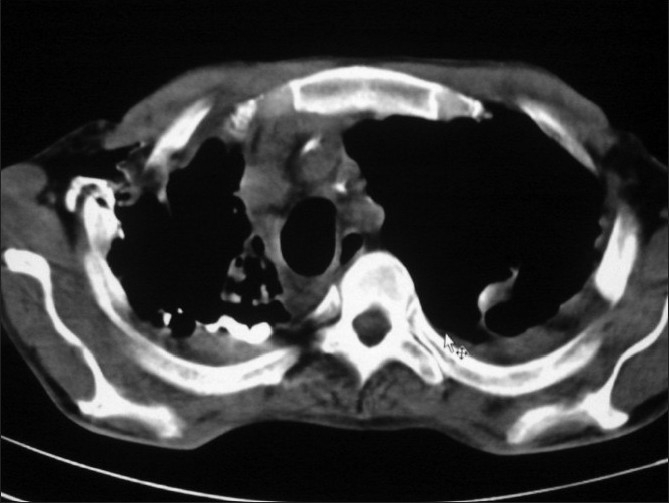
Axial chest CT demonstrates movement of dye injected in subcutaneous fistula along posterior pleural space into cavity in posterior segment of right upper lobe. From the cavity dye entered in draining segmental bronchus thus confirming the presence of bronchopleurocutaneous fistula
